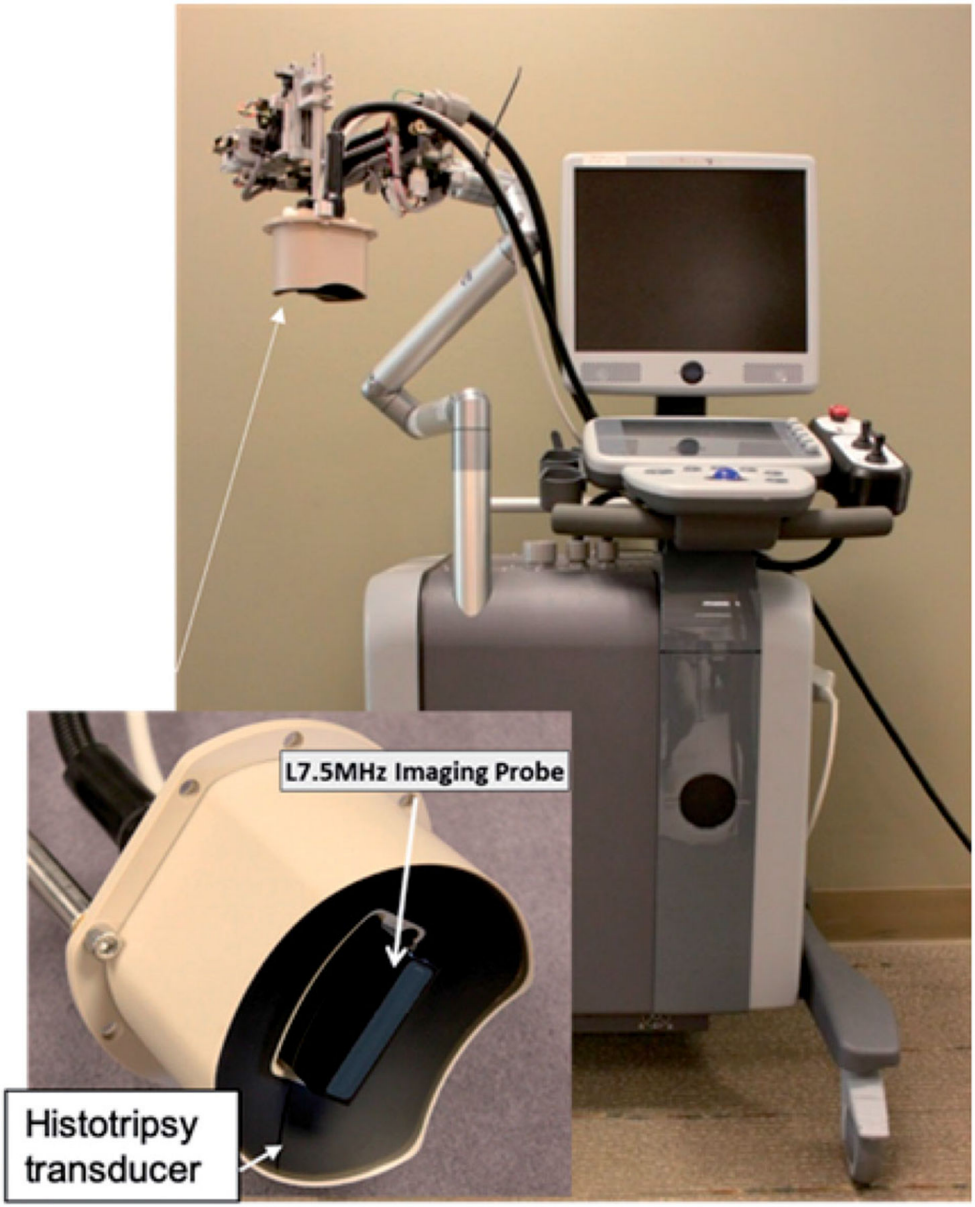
An ultrasound-guided histotripsy system that contains an ultrasound imaging engine, a histotripsy transducer with the imaging probe in the center (insert), a positioning arm that mechanically moves the histotripsy transducer.
